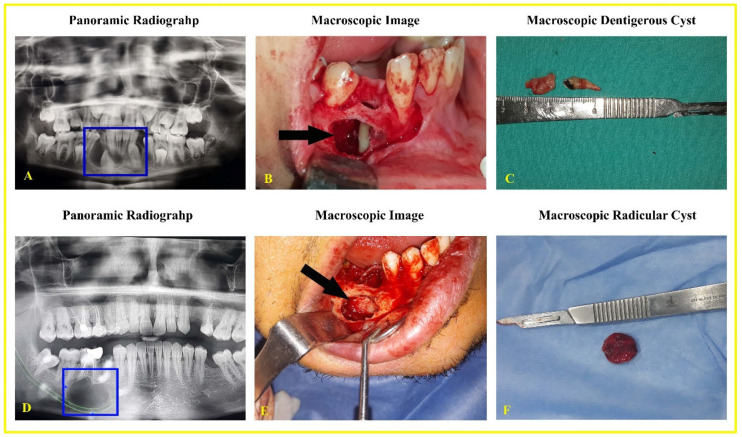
Panoramic radiography and macroscopic images of patients with odontogenic cysts. A Dentigerous cyst (A–C), radicular cyst (D–F). Cystic areas are shown in panoramic radiography (blue square) and macroscopic images (arrow).
